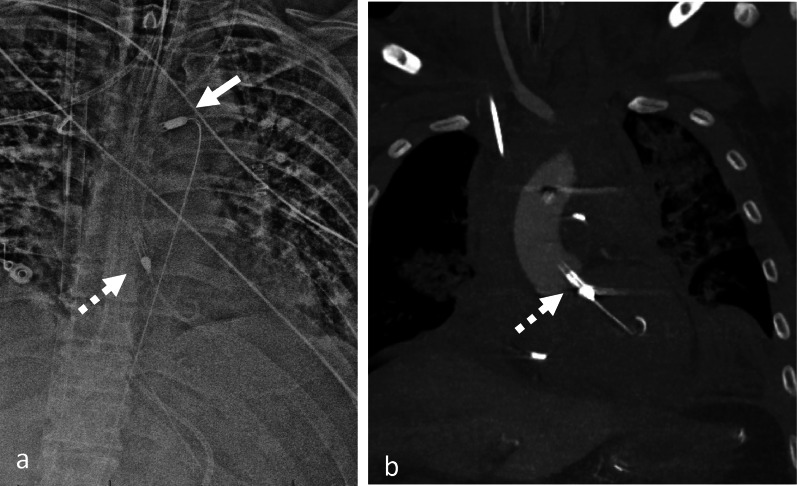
Malposition of Impella pump. AP Chest radiograph with CLAHE filter (a) with an Impella CP device with the outlet port in the aortic arch (white arrow) and the inlet port at the level of the aortic valve (dashed arrow). Contrast enhanced CT coronal image (b) at the level of the LVOT demonstrates high/proximal placement of Impella device with the blood inlet port at the level of the aortic valve. Contrast is seen only in the aorta but not in the left ventricle. This is due to backfilling from the VA ECMO cannula in the femoral artery. (CLAHE—contrast limited adaptive histogram equalization, LVOT—left ventricle outflow tract, VA ECMO—veno-arterial extracorporeal membrane oxygenation)
